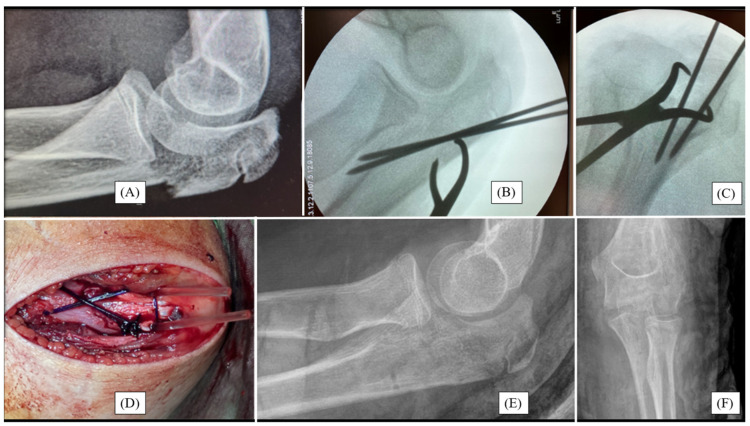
Primary X-ray of an olecranon fracture sustained by a 12-year-old boy, from lateral view (A). Fluoroscopic images after open reduction and temporary K-wire fixation (B,C). Intraoperative photograph showing the two parallel resorbable pins and a PDS compression loop (D). Postoperative X-rays demonstrate good alignment and perfect articular congruency from lateral (E) and AP (F) aspects.
